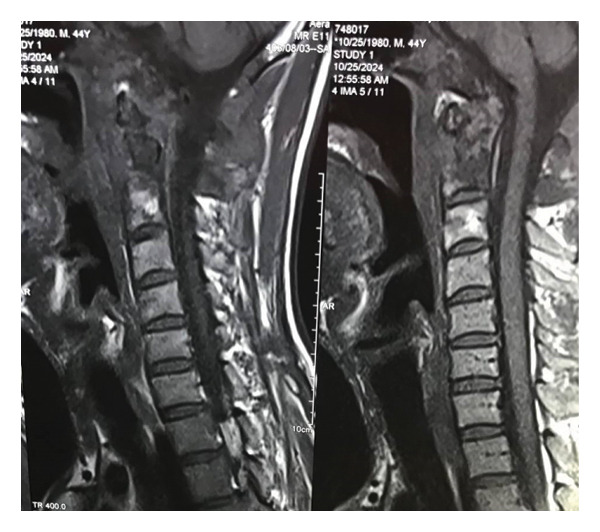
MRI of the patient showed an infiltrative enhancing lesion at the posterior skull base, affecting the right occipital bone, C1, and C2 vertebrae, with extension into the prevertebral and parapharyngeal spaces.
